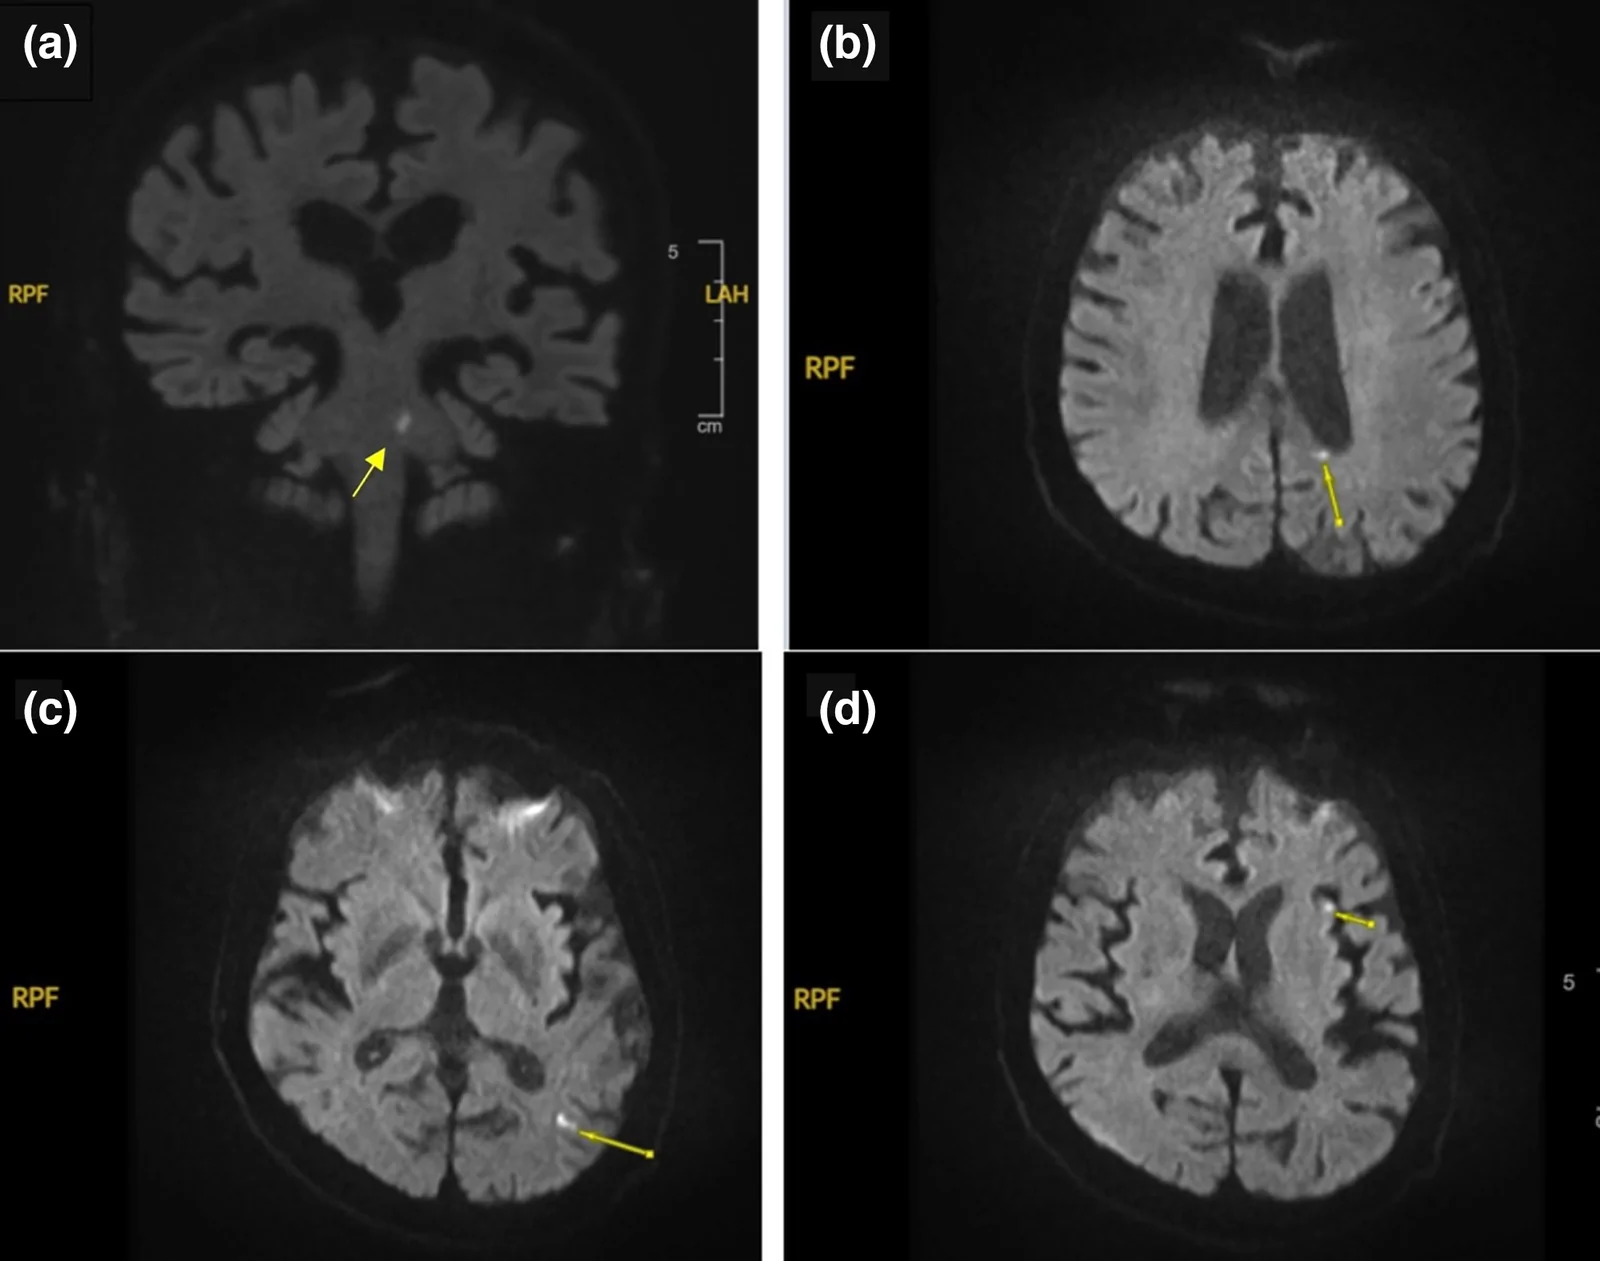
Diffusion-weighted imaging MRI of the patient’s brain showing small foci of acute infarcts in the (a) left hemi-pontine region, (b) left periventricular region, (c) left parietal lobe and (d) left insula. These possibly point to the presence of septic emboli, a minor criterion in the Duke criteria for infective endocarditis.
